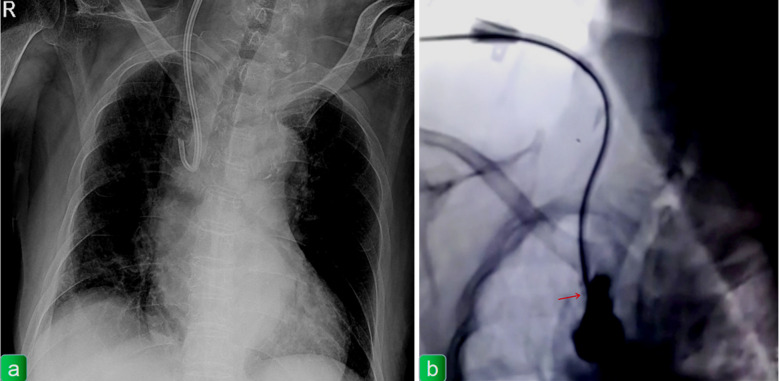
(a) Chest X-ray shows the hemodialysis catheter in the superior vena cava with its front end bent and turning back upward. (b) Digital subtraction angiography (DSA) shows contrast agent was injected into the dual lumen of the dialysis catheter and cumulative in the end of the catheter and did not spread to the periphery. Red arrows indicate the contrast agent accumulation.
